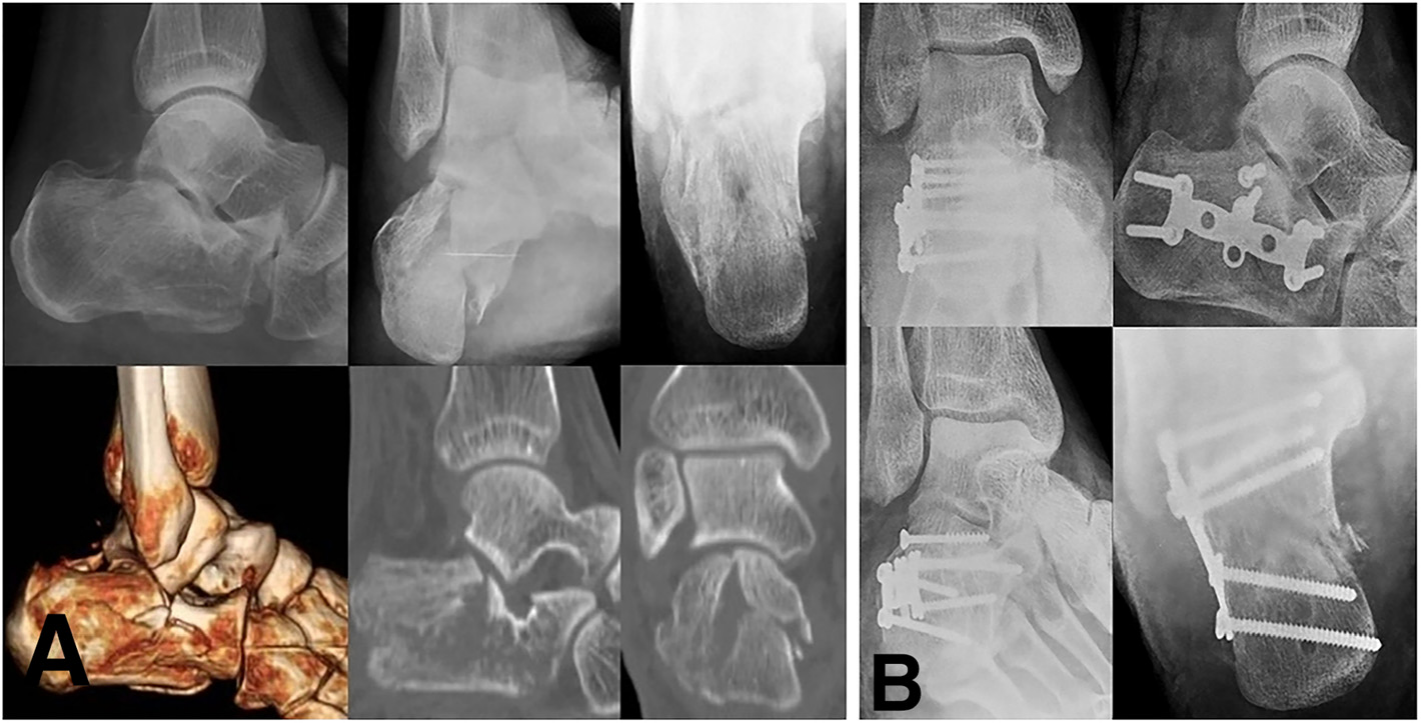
Radiologic evaluations of 52 year old male patient with Sanders type-II intra-articular calcaneal fracture treated with open reduction and internal fixation using the extensile lateral approach. (A) Preoperative plain radiographs and CT scans (B) Postoperative plain radiographs.
